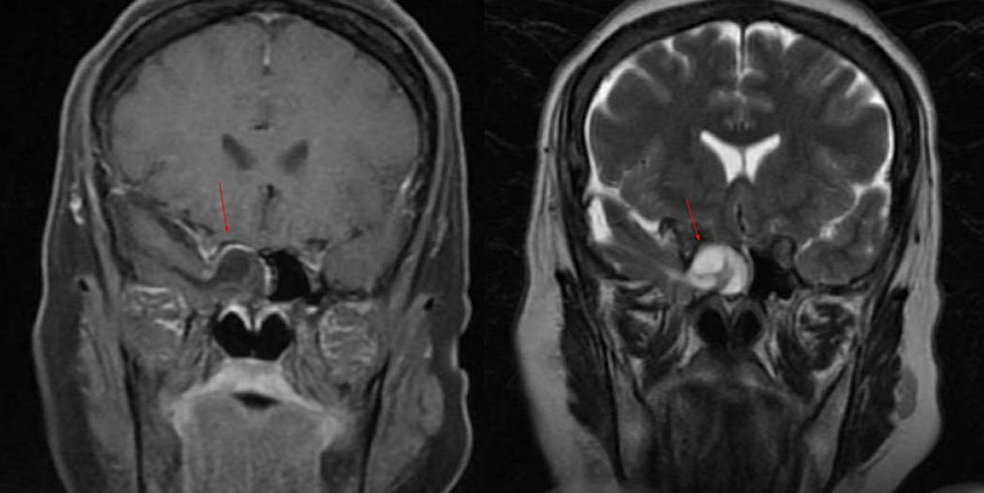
Brain magnetic resonance imaging, coronal T1 with gadolinium contrast (left), coronal T2 (right) demonstrating herniation of the meninges and portions of the inferomedial right temporal lobe through the defect into the right sphenoid sinus (red arrow)
